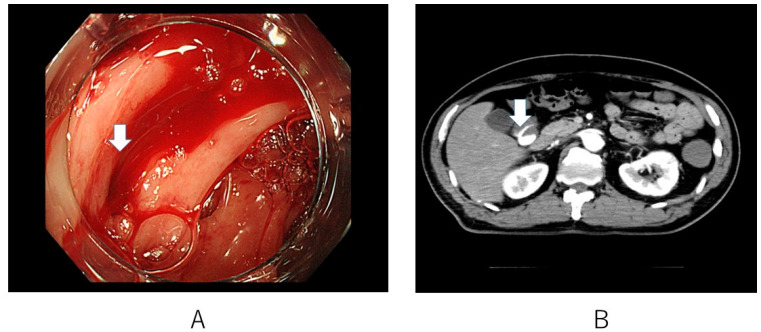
Definite colonic diverticular bleeding. (A) Bleeding from diverticula is observed with endoscopy (arrow). (B) Extraversation of the contrast medium into the colon lumen is demonstrated with computed tomography (arrow).
